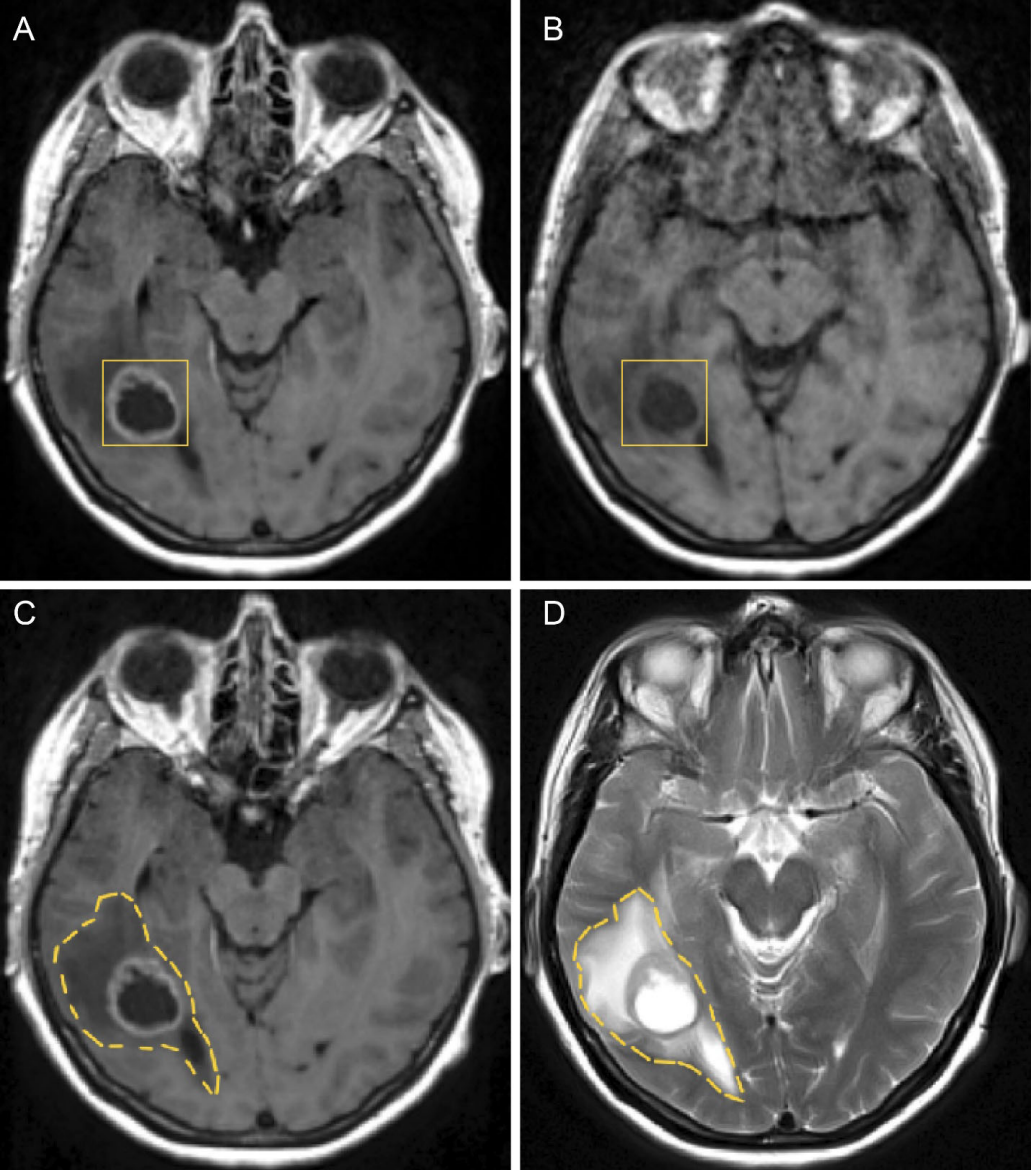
Figure showing GBM and oedema in different axial MRI scans before the first surgery: (A) GBM tumour in a T1+C, (B) GBM tumour in a T1 without contrast, (C) oedema in a T1+C and (D) oedema in a T2 weighted scan.
